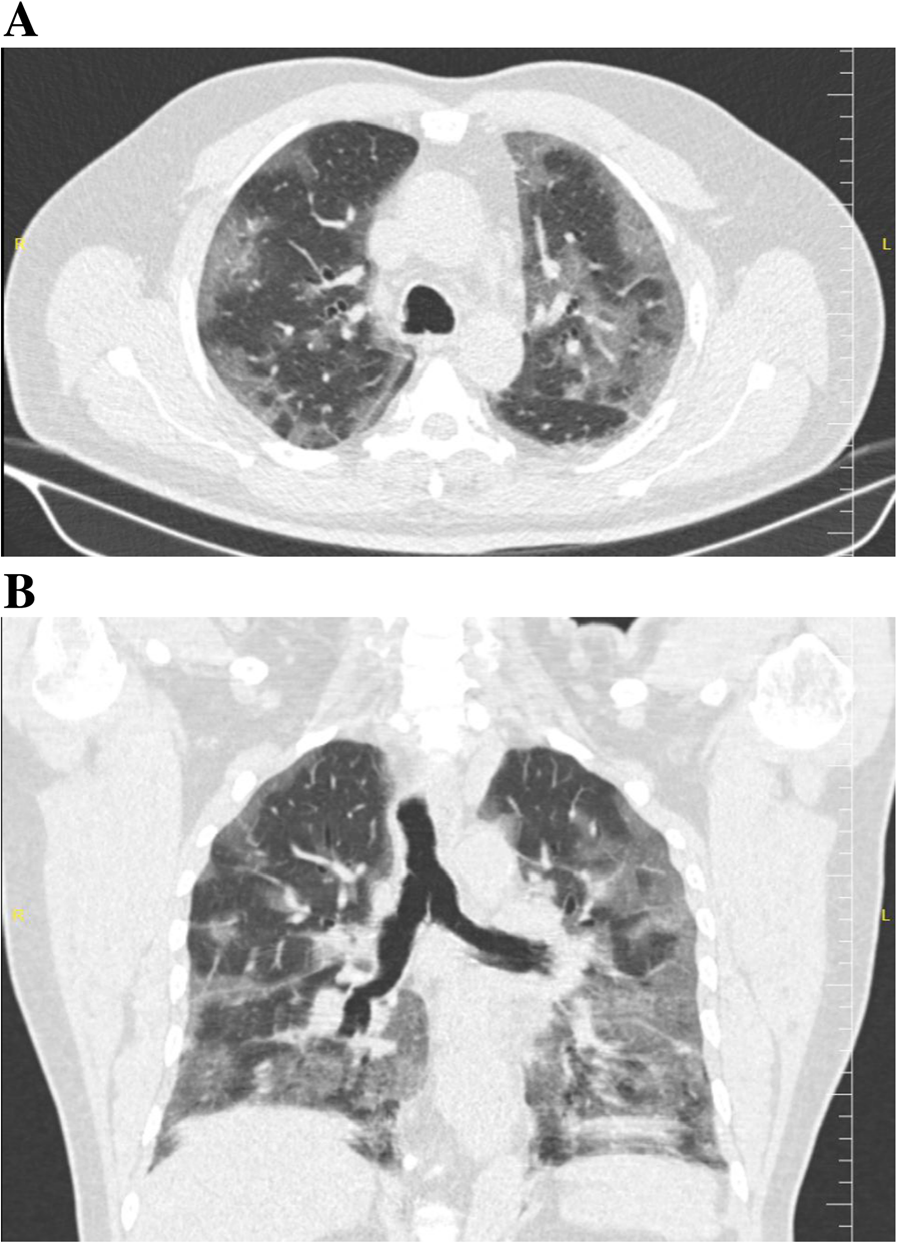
Axial unenhanced chest CT image (a) and coronal reconstructed unenhanced chest CT image (b) from a 57-year-old man with COVİD-19 pneumonia noted widespread ground-glass and consolidative opacities in both lungs with a bilateral, peripheral, and basal predominant distribution
